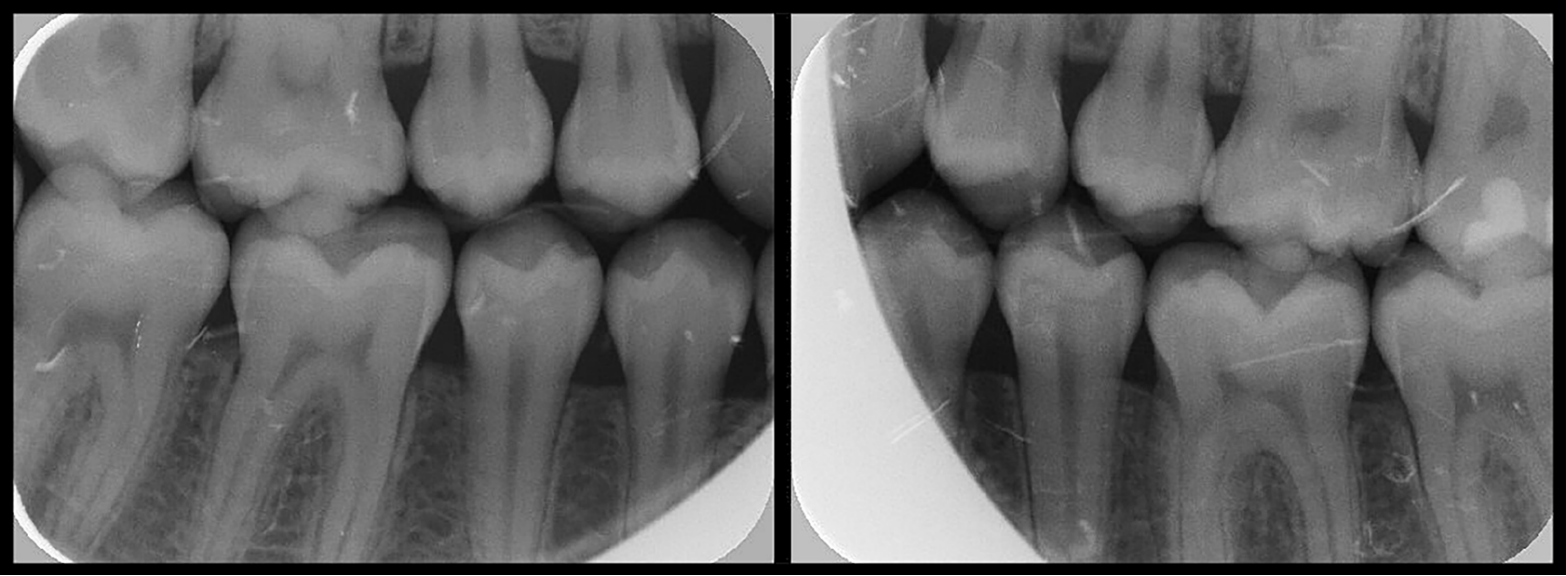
Bite-wing radiographic views of the patient in July 2005, at the age of 17 years.
